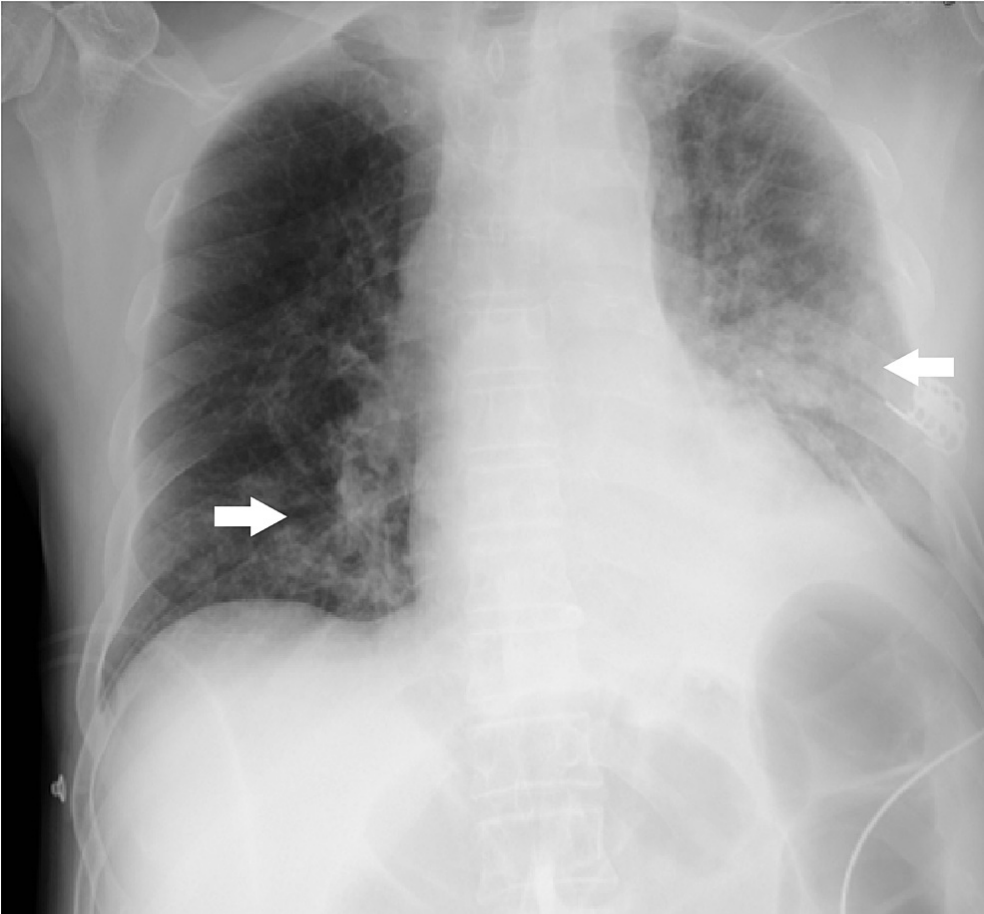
Chest X-ray showing pneumonia (white arrows).
